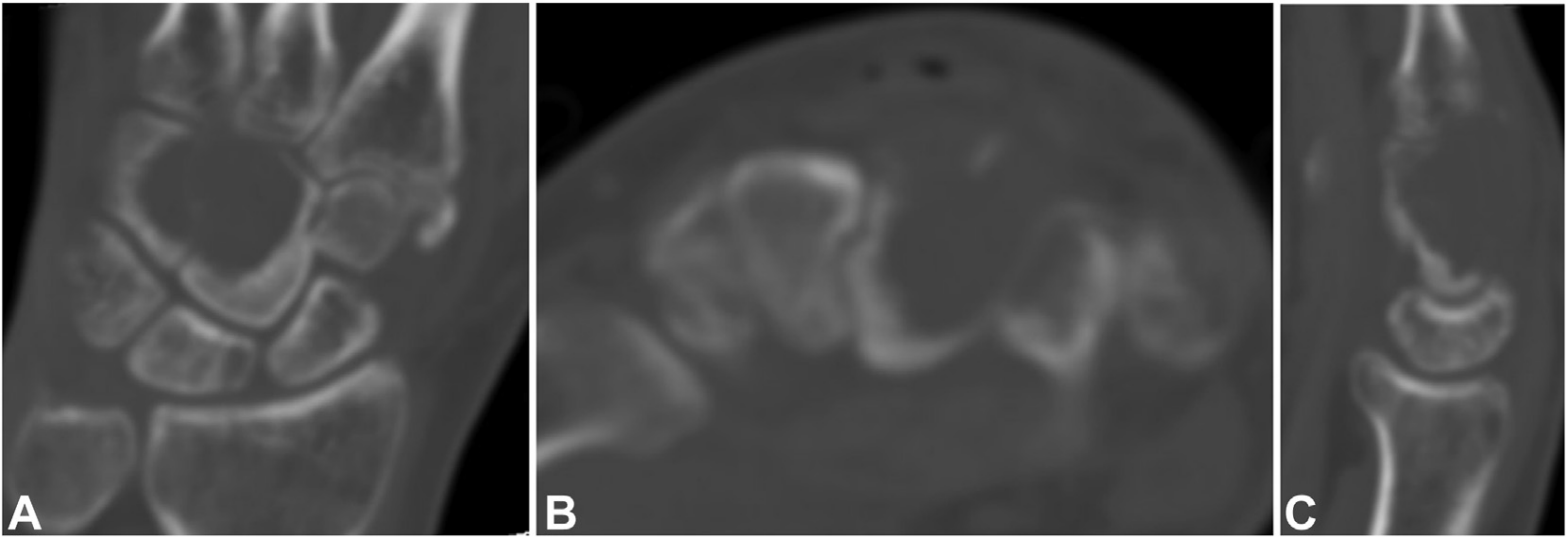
A–C Computed tomography imaging coronal, axial and sagittal sections showing a destructive osteolytic lesion of the capitate and hamate.
